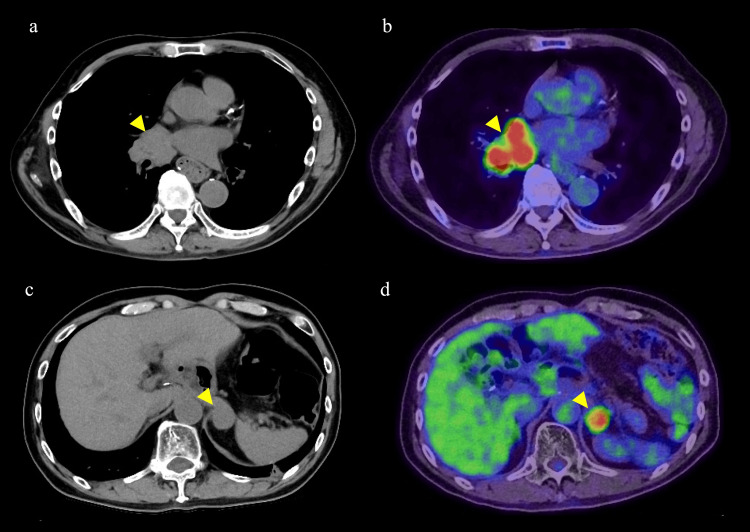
Images of distant organ recurrence. (a) CT shows swelling of the right hilar lymph nodes (yellow arrowhead). (b) PET-CT shows abnormal fluorodeoxyglucose (FDG) accumulation in the same region (yellow arrowhead). (c) CT shows a mass in the left adrenal gland (yellow arrowhead). (d) PET-CT shows abnormal FDG accumulation consistent with the mass (yellow arrowhead).
